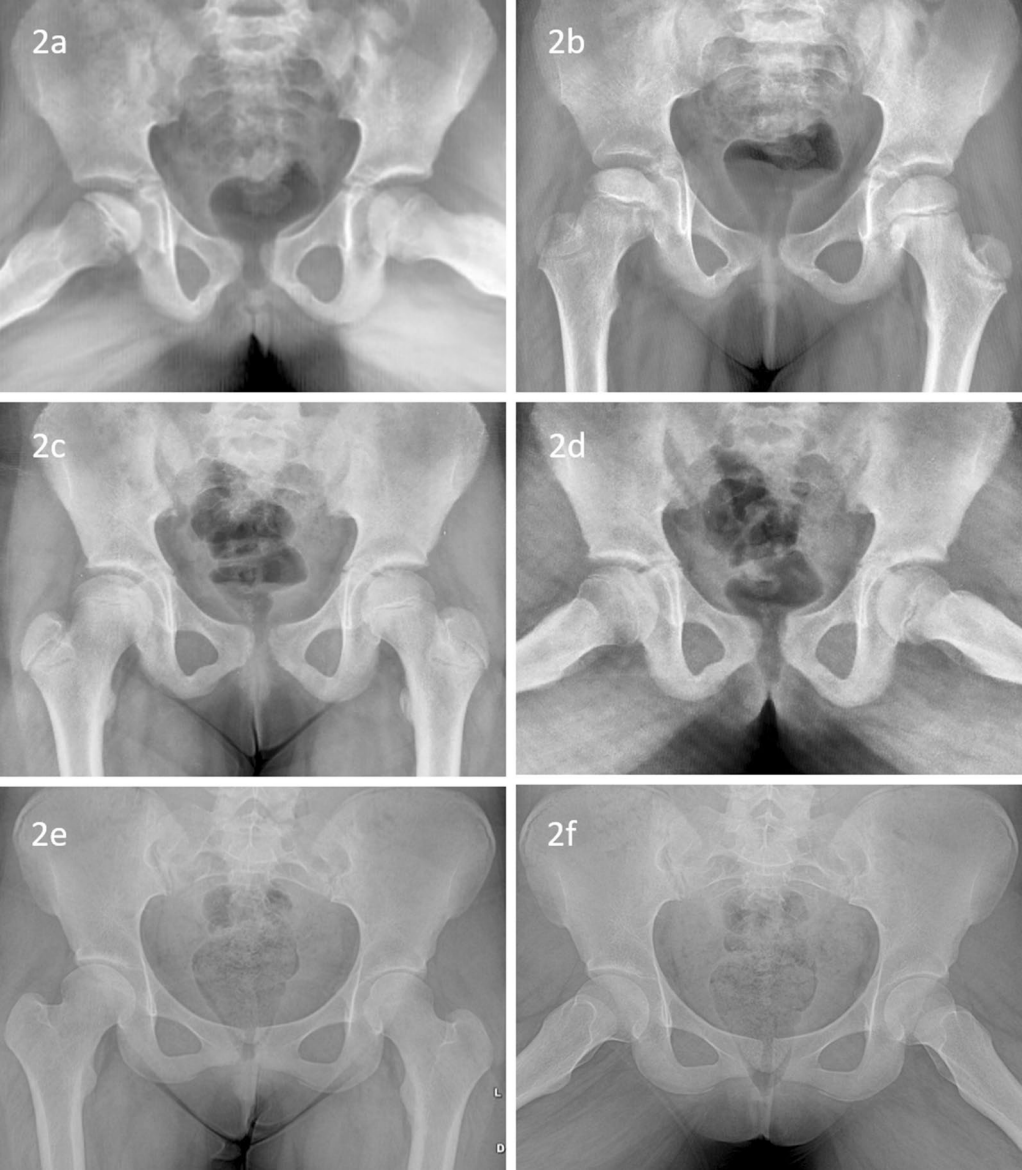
Anteroposterior (a) and frog-lateral (b) radiographs of a girl with the age of onset 6 years with right side Perthes disease stage Ib without significant extrusion. With non-operative management, the disease healed with spherical and congruent hip (c, d). At skeletal maturity, spherical congruent hip was noted (e, f)
